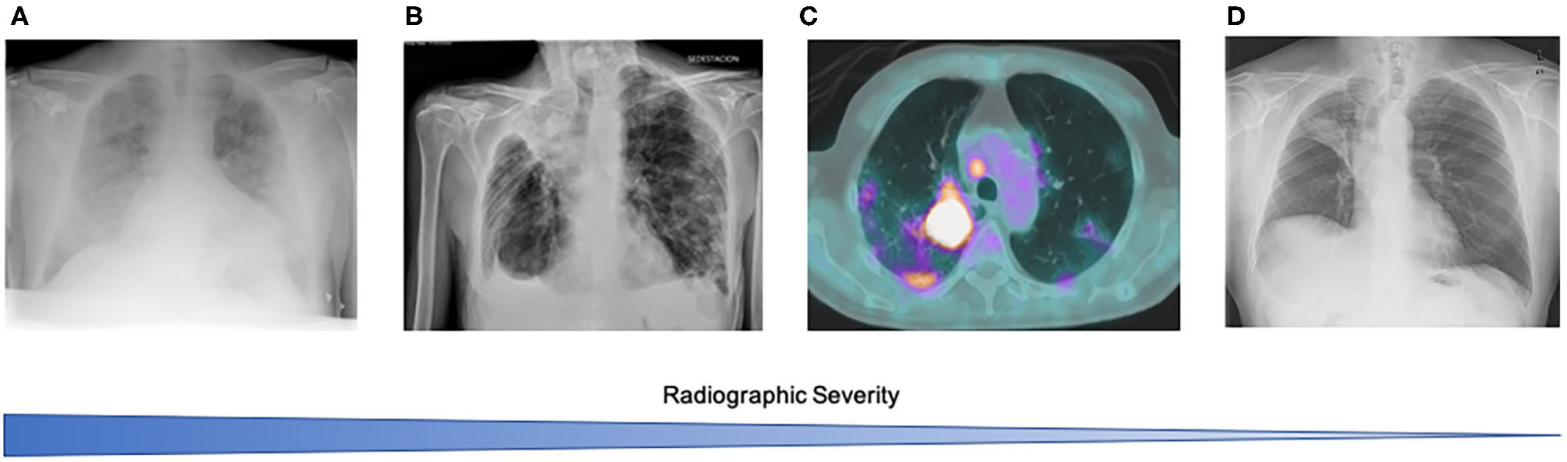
Radiological findings at the time of diagnosis of COVID-19 in patients with lung cancer. (A) Severe bilateral pneumonia in a stage IV ALK rearranged lung adenocarcinoma patient on first line alectinib; (B) Bilateral pneumonia in an 84 years-old male, stage IV lung adenocarcinoma in second line treatment with pembrolizumab. Residual findings of previous right empyema; (C) Incidental finding in a PET-CT scan performed as part of radiotherapy treatment planning in a stage IIIB locally advanced NSCLC; (D) No evidence of infiltrates in a stage IV lung adenocarcinoma patient on second line pembrolizumab. Patient was on previous antiretrovirals due to HIV. Obstructive upper right lobe atelectasis. Outcome was full COVID-19 recovery for patients in (B–D) and they could start/resume their anti-cancer treatment. Patient in (A) died from COVID-19 ARDS and massive GI bleeding.
